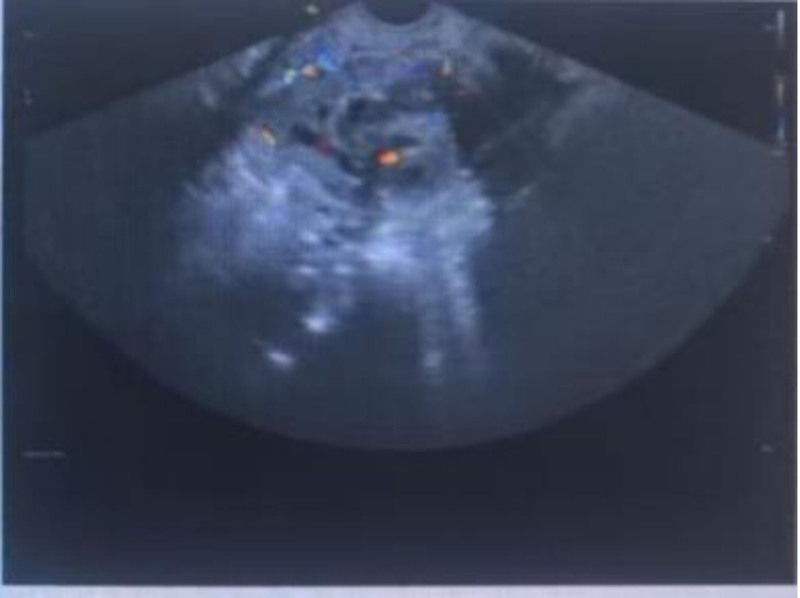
Transvaginal color ultrasound revealing a cluttered echo mass in the lower uterus with abundant blood flow signals.
